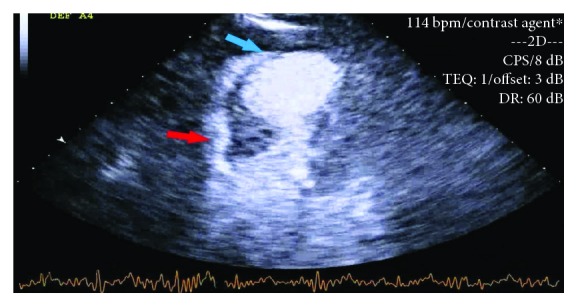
Transthoracic echo showing severely reduced ejection fraction of 30-35%, severe hypokinesis of mid to apical segment with more involvement of the mid anteroseptum, and anterior wall (blue arrow) with basal hyperkinesia and basal asymmetric hypertrophy of the septum (red arrow).
